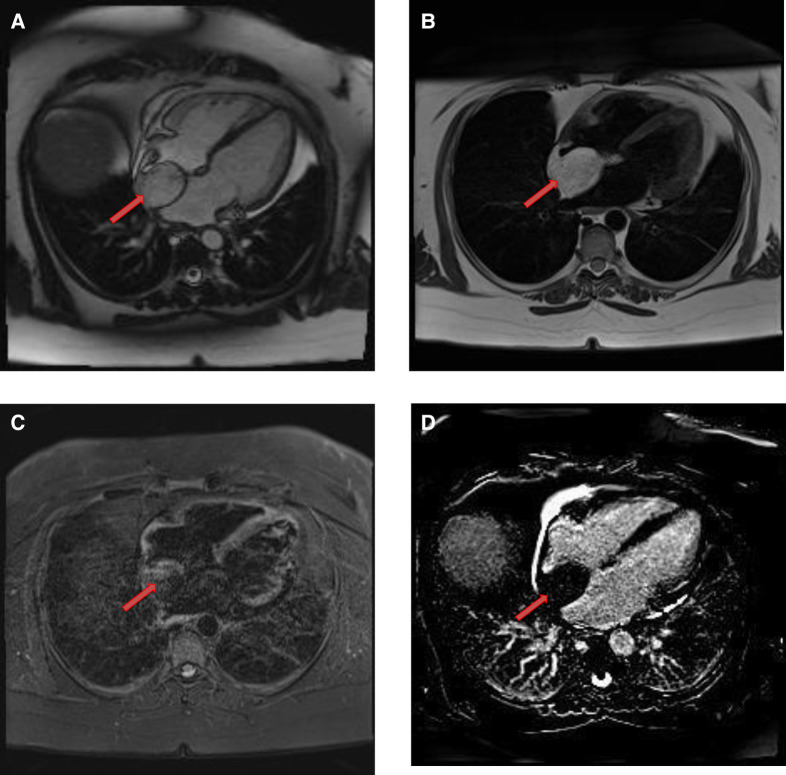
A 50-year-old female with intra-atrial septal lipoma. (A) This mass has signal intensity (high) as surrounding fat on axial T2-weighted, dark blood, double inversion recovery fast spin echo images (red arrow). (B) This mass is suppressed on axial triple inversion recovery images (red arrow). (C) Complete absence of contrast agent uptake on late gadolinium enhanced images (red arrow).
